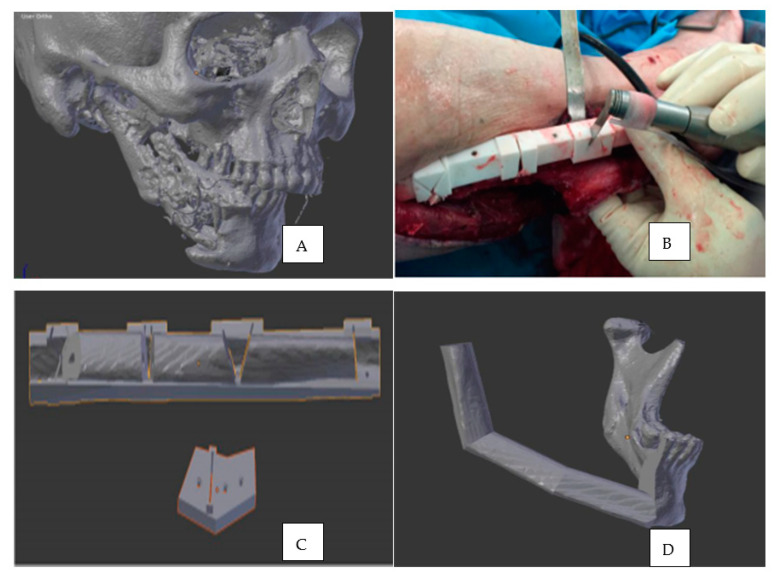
(A) CT of skull with osteoradionecrosis (B) 3D printed surgical guides used to guide osteotomies to obtain fibula from patient’s leg. (C) Surgical guide with harvested bone. (D) Surgical reconstruction of the jaws using scanning and 3D modelling. Image reprinted with permission from Ganry L et al. [99].
